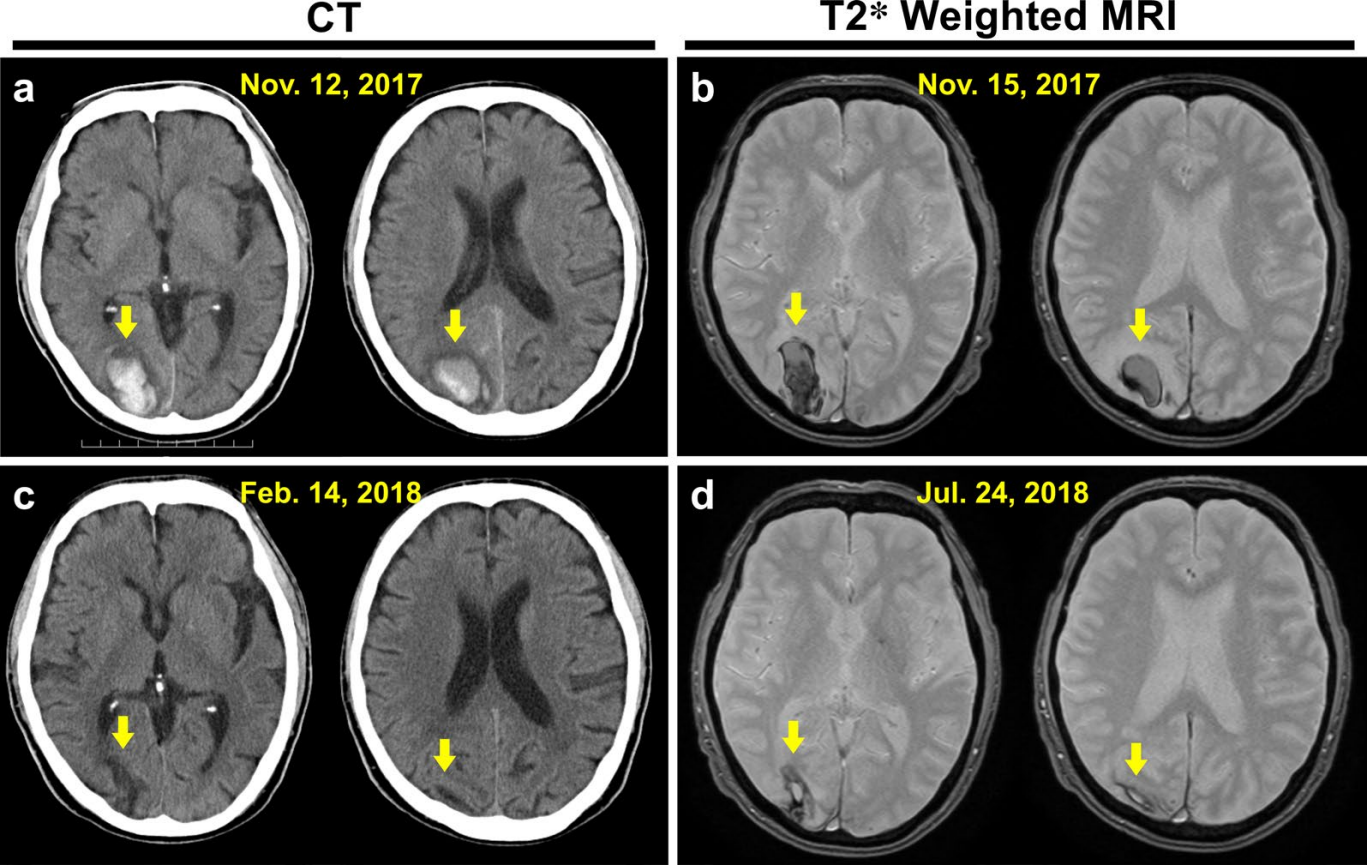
Computed tomography (CT) of the brain on 12 November 2017 (a) and 14 February 2018 (c), and T2*-weighted magnetic resonance imaging (MRI) of the brain on 15 November 2017 (b) and 24 July 2018 (d). Two different slices were included on each date. High density on the CT (a yellow arrowhead) and low intensity of the peripheral zone of the lesion on the T2*-weighted MRI (b yellow arrowhead) revealed cerebral hemorrhage of the right occipital lobe. Three months after the stroke, the high-density area disappeared, and the small low-density area remained on the brain CT (c yellow arrowhead). The low-intensity lesion decreased in size 8 months later on the brain T2*-weighted MRI (d yellow arrowheads)
